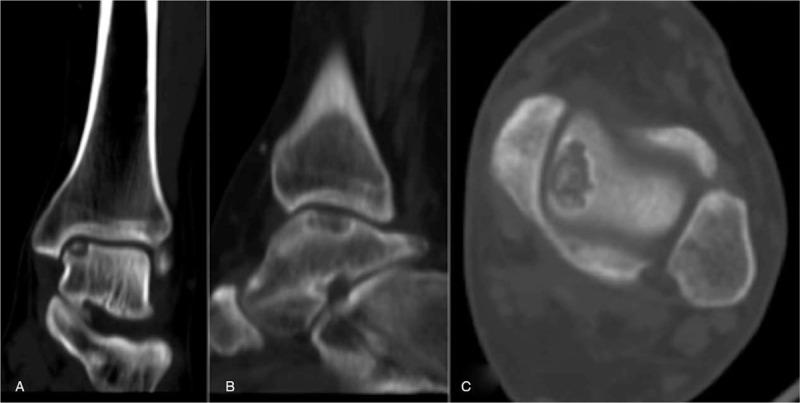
The preoperative computed tomography (CT) images of the left ankle joint present the 15.09 × 9.54 × 5.72 mm low density cystic defect area in the talus from the coronal (A), sagittal (B), and transactional (C) views.
